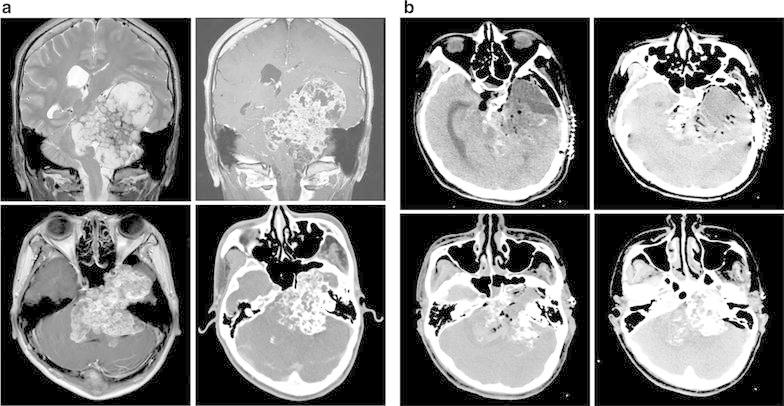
a. Preoperative radiologic imaging of patient 1 with PSH. Huge size (8.1 cm) extraaxial calcified mass over left middle cranial fossa and posterior cranial fossa is compressing brainstem. This mass originates from petrosal apex and displaces midbrain, pons, and middle cerebellar peduncle to right side. (*Clockwise direction*; T2WI coronal, T1W enhance coronal, CT with enhance, T1W enhance axial). b. Postoperative (POD 20) CT scan showing residual calcified mass adjacent to brainstem and hemorrhage, edema in brainstem
